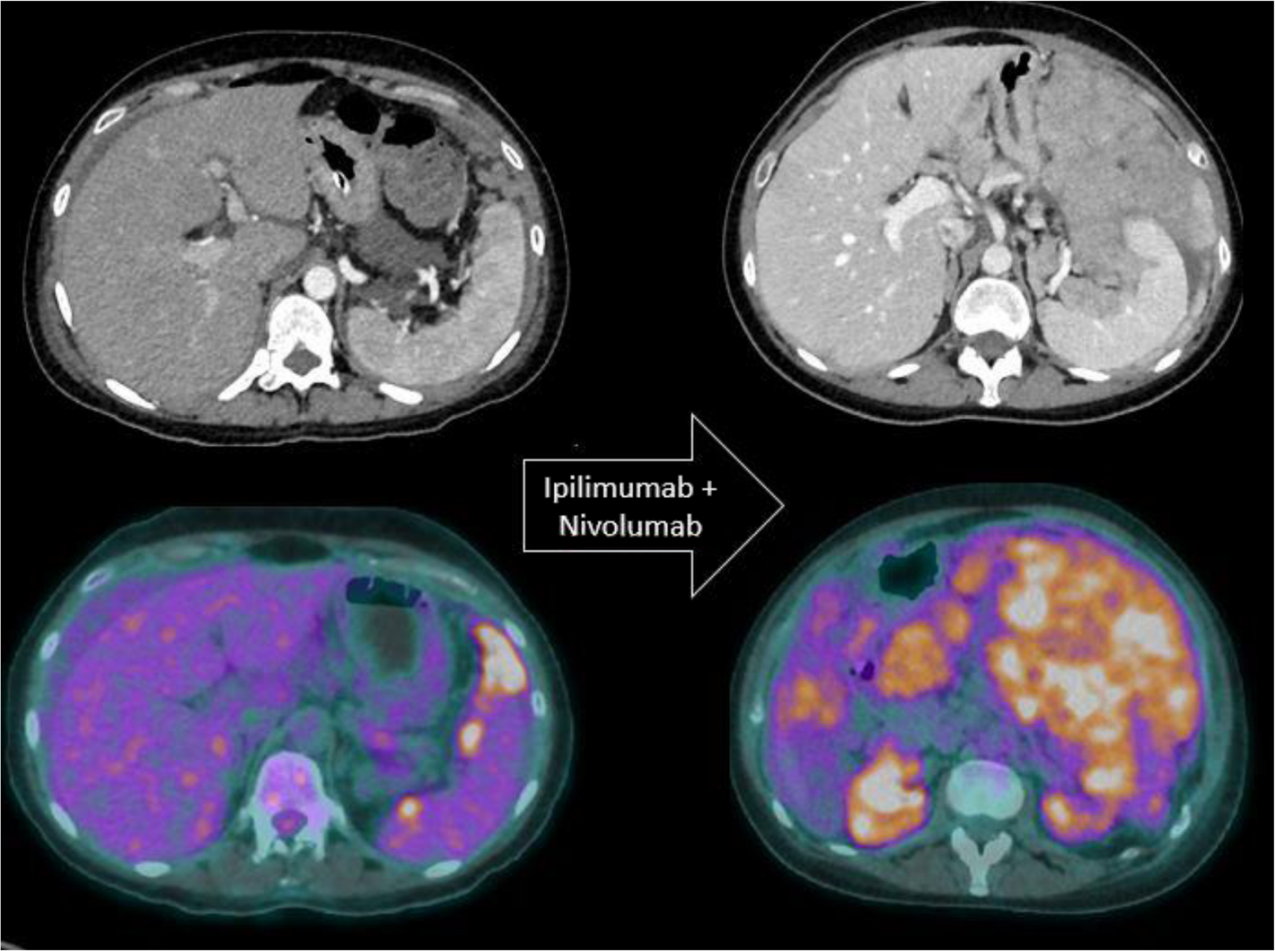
Paradoxical acceleration of tumour growth kinetics in a patient with metastatic melanoma treated with ipilimumab and nivolumab. Baseline axial CT image and corresponding ^18^F-FDG PET/CT image show few perisplenic peritoneal metastatic implants. Two months after the initiation of immunotherapy, both imaging modalities show a dramatic increase in peritoneal metastases.
